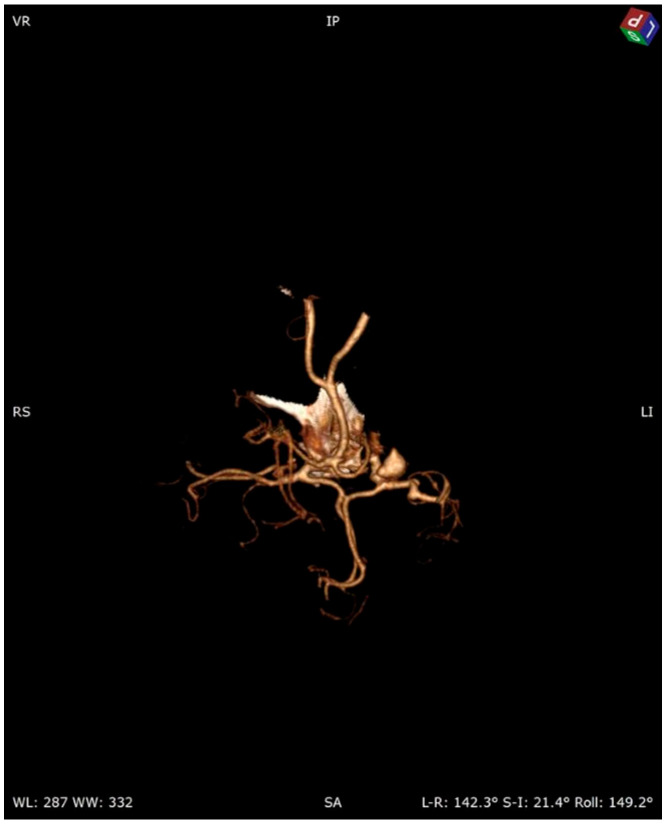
Cerebral 3D CT angiography.
